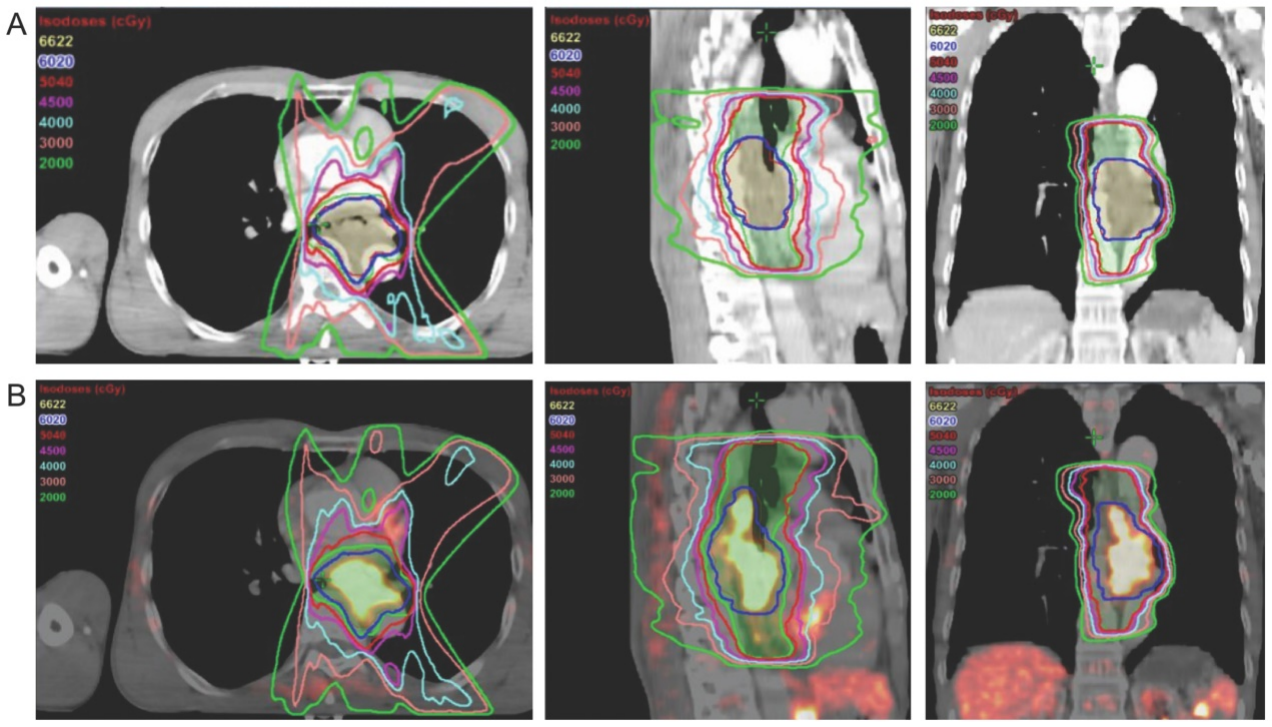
Radiation treatment plan for a 57-year-old male patient with lower esophageal cancer based on (A) contrast-enhanced CT (tumor length, 4 cm; GTV volume, 39.32 cm^3^); and (B) CT + FAPI ×20% (tumor length, 7.5 cm; GTV volume, 41.73 cm^3^). Adapted with permission from 46, copyright 2021 Elsevier.
